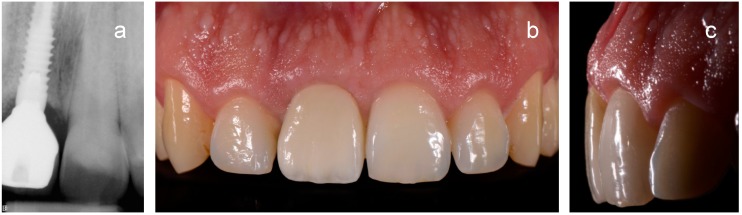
Definitive restoration of the first case after three years: radiographic (a) and clinical appearance (b,c).
